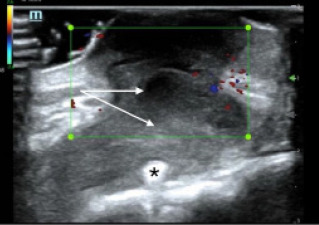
A point-of-care ultrasound of the anterior chest wall with color flow Doppler demonstrating a heterogeneous structure (arrows), extending to the driveline (star) without significant internal color flow.
